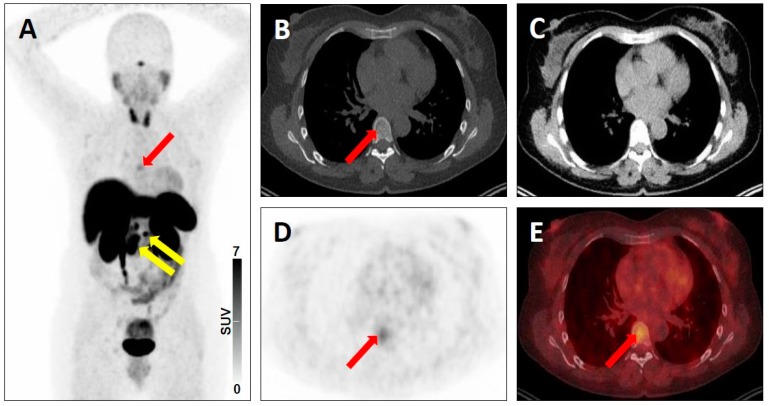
Equivocal uptake in bone lesion on SSTR-targeted positron emission tomography (PET)/computed tomography (CT). ^68^Ga-DOTATATE PET/CT for restaging in a 52-year-old woman with a history of ileal neuroendocrine carcinoma. Whole-body maximum intensity projection image showing multiple metastases to lymph nodes with clearly pathologic radiotracer uptake (yellow arrows), consistent with SSTR-RADS 5 lesions and a faint osteolytic bone lesion (red arrow) (A). Axial low-dose bone-window CT image (B), soft-tissue window CT image (C), PET image (D), and fused PET/CT image (E) further show this faint osteolytic bone lesion on anatomic imaging with equivocal uptake (red arrows), consistent with a SSTR-RADS-3B lesion.
